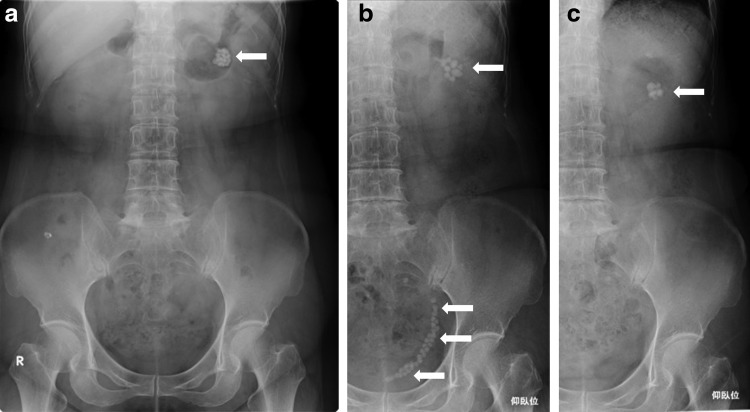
KUB image (a) KUB image 8 years before the reference to our hospital reveals many spherical shaped calculi at the upper pole of the left kidney (*arrow*). (b) KUB image at the time of visiting our hospital reveals many spherical shaped calculi at the upper pole of the left kidney and beaded calculi that occupied left lower ureter (U2–U3) (*arrows*). The number of the calculi at the upper pole of the left kidney is decreased as compared with that of KUB image 8 years before. (c) KUB image 1 month after the first visit showed all ureteral calculi are excreted (*arrow*). KUB, kidney, ureter, and bladder radiograph.
